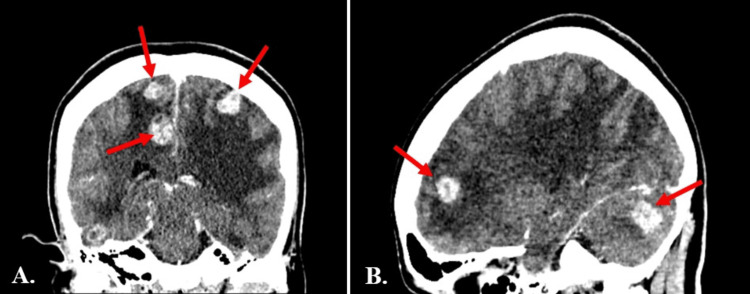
Contrast-enhanced computed tomography of the brain showing (A) coronal and (B) sagittal sections demonstrating multiple enhancing intracranial lesions with surrounding vasogenic oedema and moderate mass effect (arrows).
